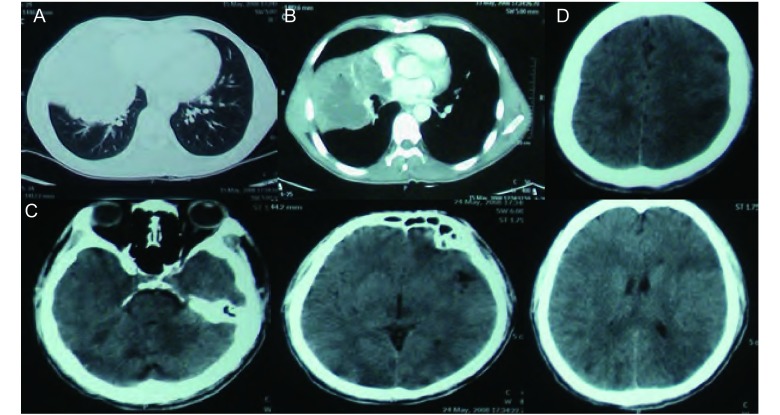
典型的术前肺部CT扫描片和典型的术后头部CT扫描片。A：肺窗显示右肺中下叶肺门处巨大肿物；B：纵隔窗显示右肺中下叶巨大团块状软组织影，侵犯心包；C：显示阻塞的基底动脉末端引起基底动脉尖综合征；D：显示左侧大脑中动脉分支闭塞引起顶叶脑栓塞 The classical CT scan of lung before operation and cephalosome after operation. A: Chest CT scan of lung shows huge tumor of the right lung; B: Chest CT scan of mediastinum shows huge conglomeration of parenchyma in the right lung.It encroach pericardium; C: It shows that obstructed basilar artery lead to TOBS; D: It shows cerebral embolism of parietal lobe because the ramus of the left middle cerebra, artery is obturated
